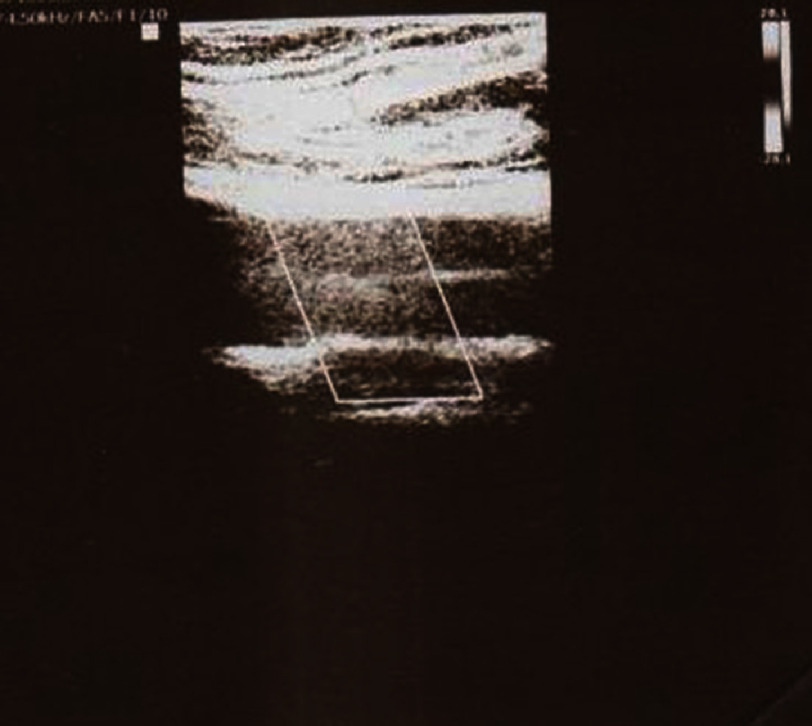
Doppler ultrasound showing non-compressible saphenous vein due to thrombosis.
